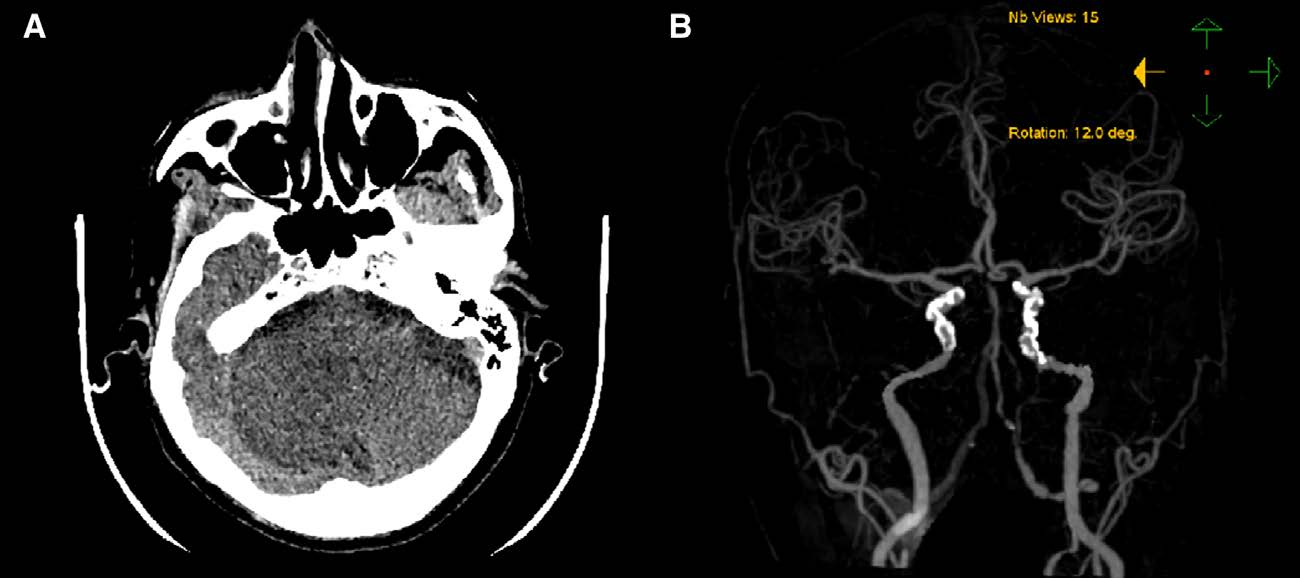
A 70-year-old elderly man with MCI including in our study, presented with a sudden disturbance of consciousness for 5 h. (A) Cranial CT suggested massive cerebellar infarction on the right side at admission. (B) Cranial CTA suggested basilar artery occlusion. The patient was conscious on the 1st day after EVT, but lost consciousness and entered a deep comatose state on the 2nd day. The family refused further treatment and was discharged soon thereafter. CT = computed tomography, CTA = computed tomography angiography, EVT = endovascular treatment, MCI = massive cerebellar infarction.
